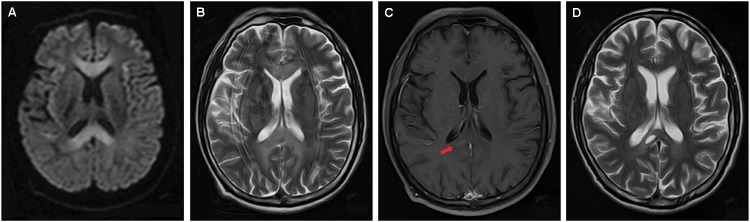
Axial images of brain MRI performed 7 days (A–C) and 22 days (D) after onset. MRI performed 7 days after onset revealed symmetrical and bilateral hyperintense lesions on diffusion-weighted imaging (DWI) (A), and T2-weighted imaging (B) throughout the entire corpus callosum (CC). Extracallosal lesions could also be seen (A,B). T2-weighted imaging had a considerable amount of distortion due to motion because the patient couldn’t cooperate well (B). Gadolinium enhancement could be seen in part of splenium (C, arrow). 22 days after onset, follow-up MRI showed only mild remaining of the formerly impressively hyperintensity on D2-weighted imaging, and the formation of necrosis in the splenium (D).
